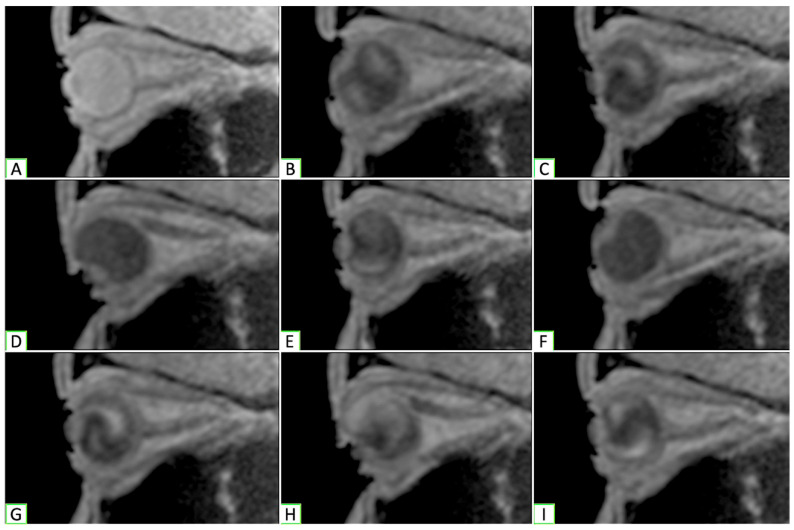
Dynamic orbital MRI (Vedio S1) cuts from each position of gaze analyzed. (A,C,E,G,I) Primary; (B,F) upgaze; (D,H) downgaze.
